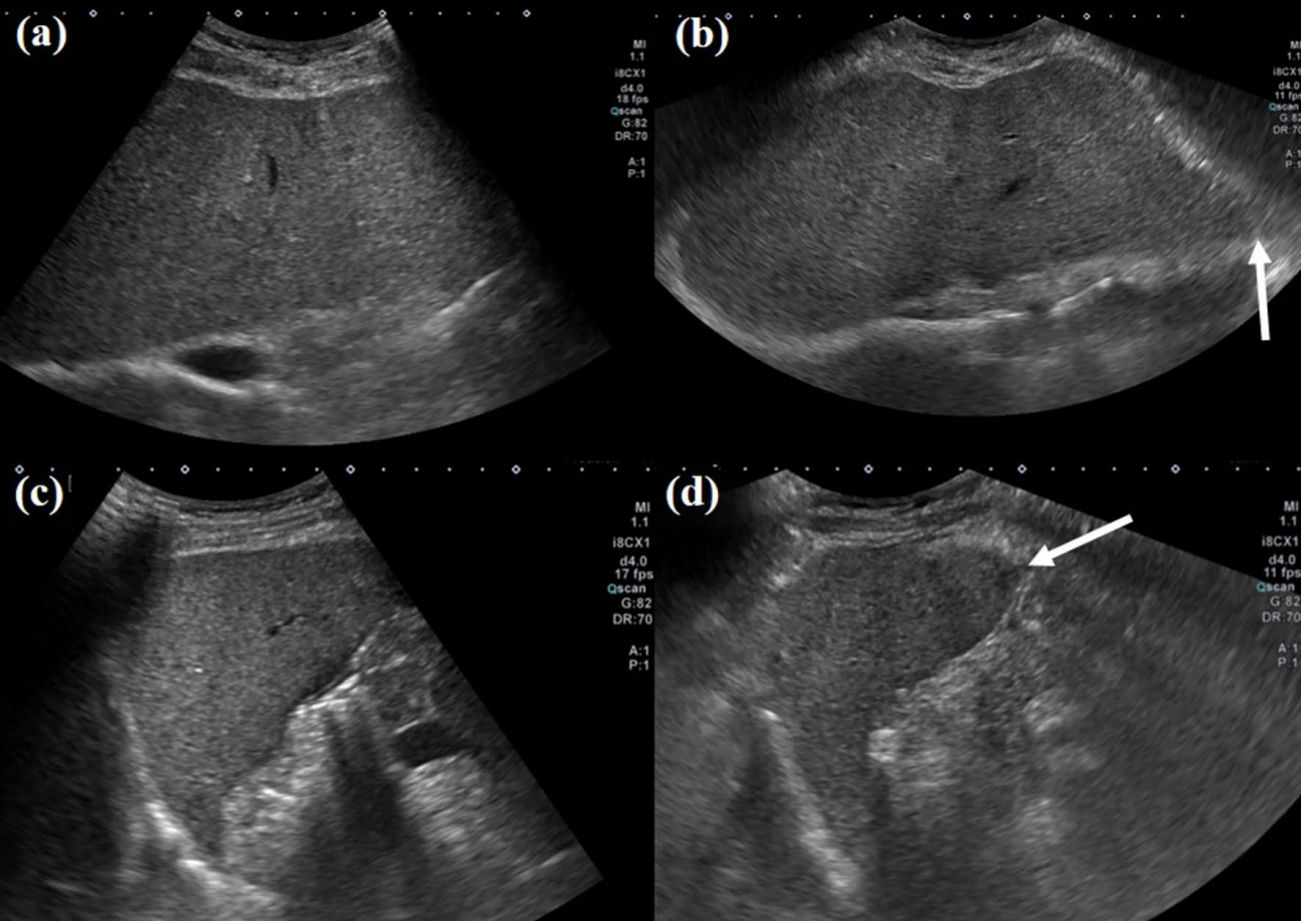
Figure 1. Ultrasound (US) images acquired from a 61-year-old male patient with a history of prior gastric cancer surgery. a Transverse liver left lobe scan using a routine view mode, showing limited coverage that does not include the left lateral segment tip of the liver. b Transverse liver left lobe scan using a wide view scan mode, providing complete coverage of the border of the liver left lobe, including the left lateral segment tip (arrow). c Sagittal liver left lobe scan using a routine view mode, not displaying the left lateral segment tip of the liver. d Sagittal liver left lobe scan using a wide view scan mode, including coverage of the left lateral segment tip of the liver (arrow)
